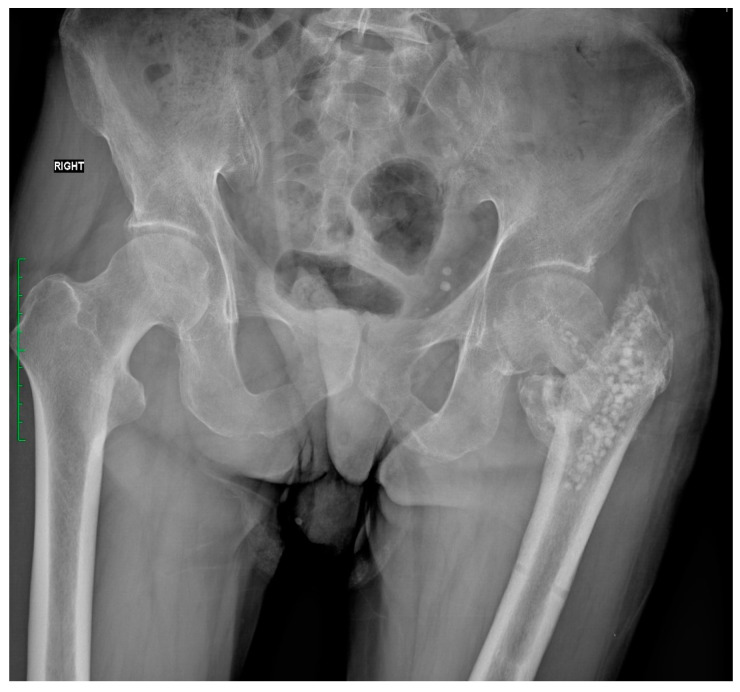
X-ray after surgery. Procedure to remove, fuse, and fill bone defects with absorbable calcium sulfate antibiotic carrier with Gentamicin.
